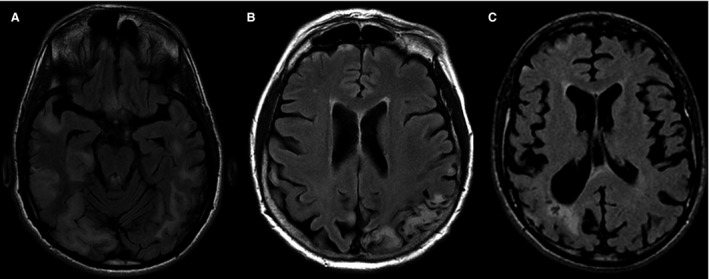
Conventional neuroimaging of three representative MELAS patients. (A) A 28‐year‐old patient harboring the 3243A > G/MT‐TLA1 mutation and presenting the typical features of stroke‐like lesions (SLL). Axial FLAIR T2‐w shows cortical‐subcortical lesions prevalently in the temporo‐parieto‐occipital regions. (B) A 59‐year‐old female with T10191C ND3 mutation (#15) was asymptomatic at MRI scan and had the last stroke‐like episode (SLE) more than 3 months previously. She presented occipital epilepsy from childhood with several episodes of status epilepticus. Axial FLAIR T2‐w showed gyral necrosis affecting the cortex and juxta cortical parieto‐occipital white matter with partial gyral signal suppression on T2/FLAIR sequences, a feature referred to as “black toenails” sign. (C) A 30‐year‐old male with T3271CmtDNA mutation (#19) was asymptomatic at scan evaluation. One month before the scan, he had an SLE that clinically presented with epileptic seizures. Axial FLAIR shows a chronic lesion with focal brain volume reduction in the right parieto‐occipital white matter, characterized by T2 FLAIR hyperintensity with secondary ventricular dilatation and foci of cavitary white matter necrosis.
